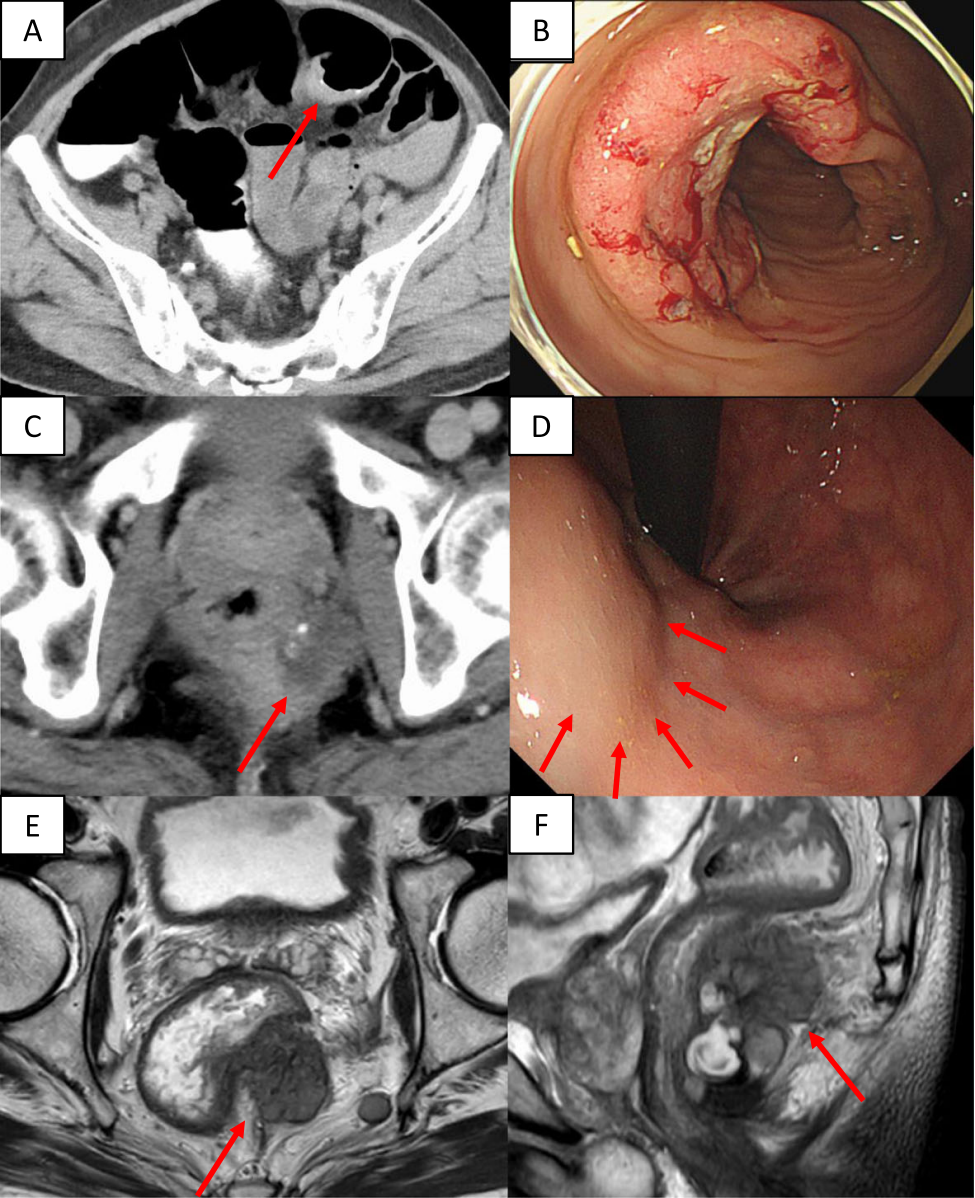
Image of the sigmoid colon cancer and rectal NET. A contrast-enhanced CT (a) and a colonoscopy (b) showed a sigmoid colon cancer. A contrast-enhanced CT (c) and a colonoscopy (d) showed a rectal NET. An MRI (e axial views, f sagittal views) showed the rectal tumor definitely developed from the rectum. Each arrow shows the lesion
